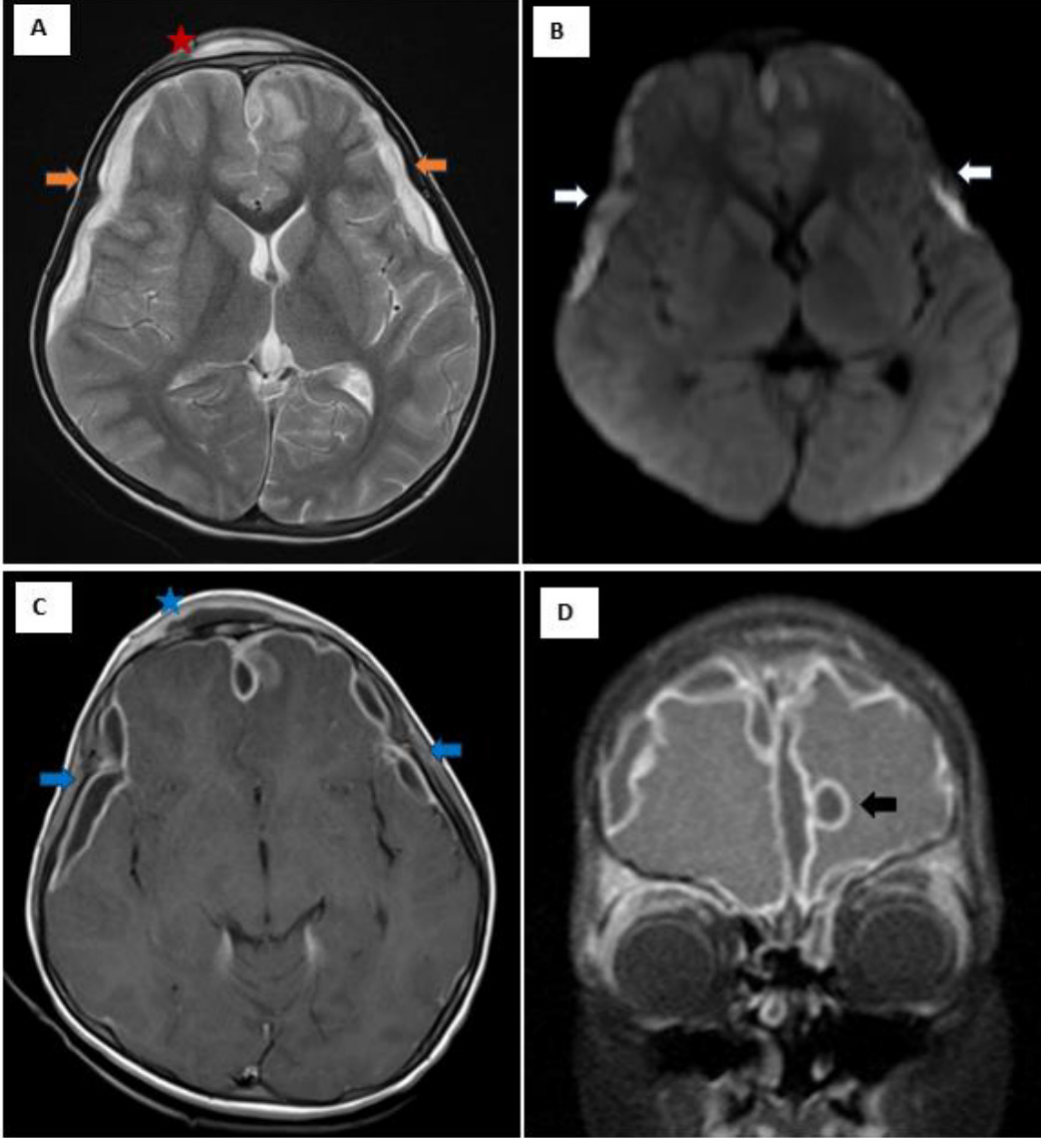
MRI Images of an 8-year-old boy with Pott’s puffy tumour showing: A – Axial T2 images showing subdural collections (orange arrows) and a subperiosteal abscess (red star) overlying the frontal bone predominately on the right side; B – DWI images showing diffusion restriction of the collections (white arrows); C – Axial contrast-enhanced T1 sequence showing peripherally enhancing subdural collections (blue arrows) and sub-periosteal abscess (blue star); D – Coronal contrast-enhanced T1 sequence showing extensive peripherally enhancing subdural collections with a parafalcine collection causing indentation of the left frontal lobe (black arrow)
